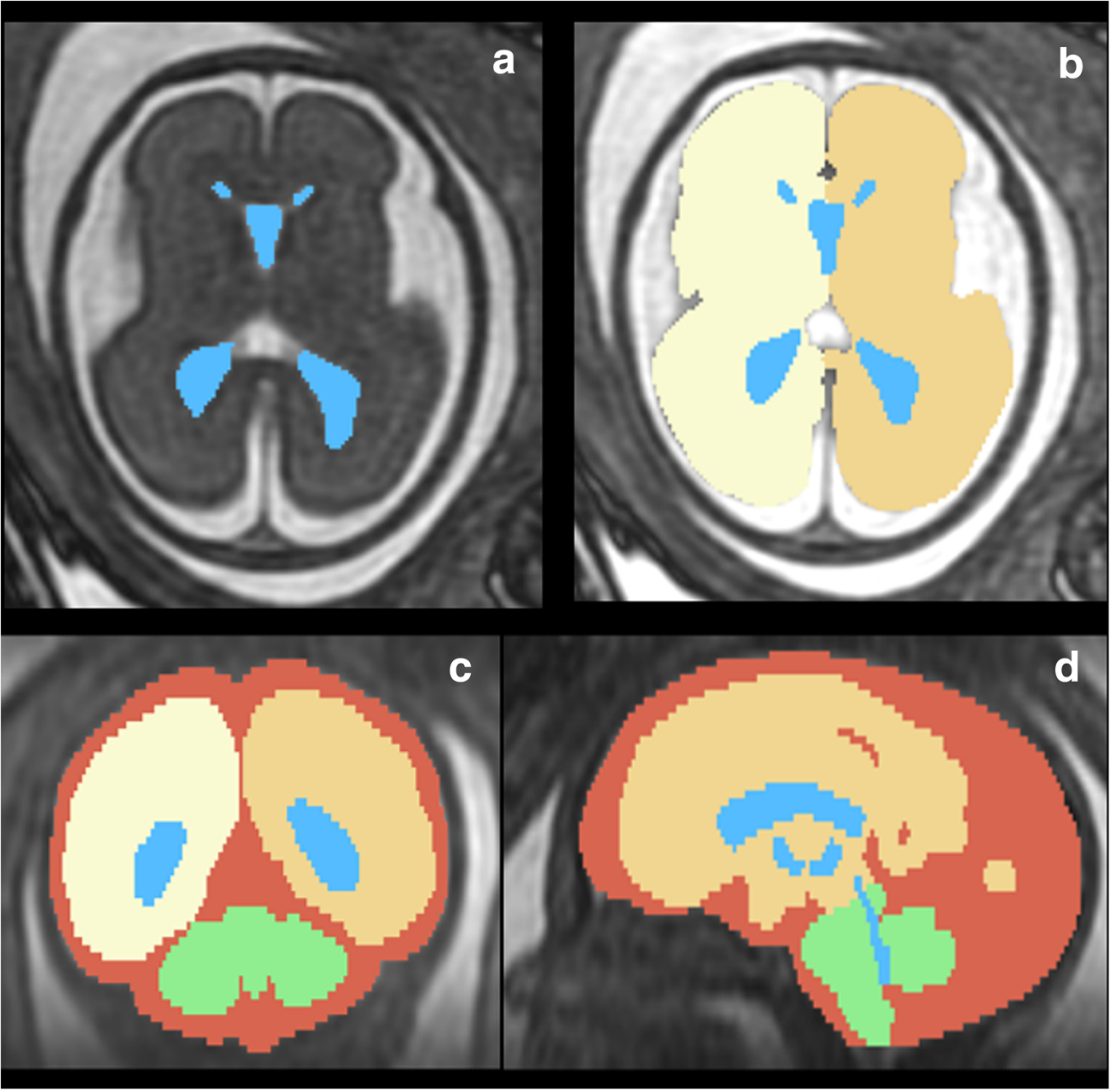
In utero MR imaging of a normal control fetus at 26 gestational weeks showing the regions of interest segmented for volume measurements; (a) Axial 3D FIESTA showing the ventricular system segmented in blue and (b) cerebral hemispheres represented by cream and yellow. (c) Reconstructed coronal and (d) sagittal images with the extra-axial spaces shown in red and the cerebellum and brain stem in green
